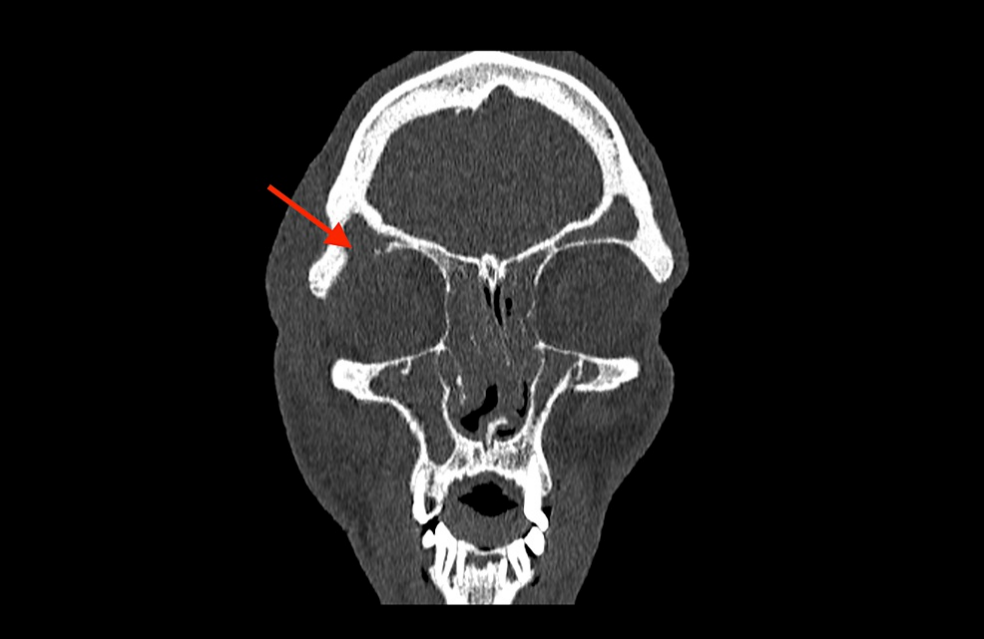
Computed tomography (CT): a coronal reconstruction with bone windows showing complete opacification of bilateral maxillary, ethmoid and frontal sinuses with a defect in the inferolateral aspect of the right frontal sinus
